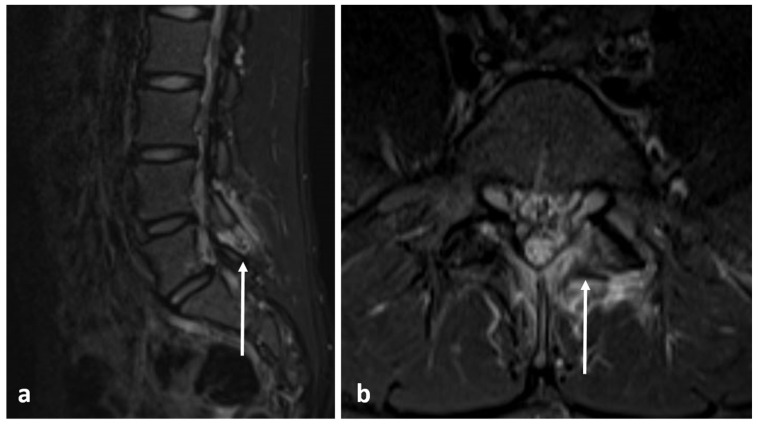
Sagittal (a) and axial (b) STIR images demonstrating the same osteoid osteoma showed in Figure 3a,b (white arrows). High STIR signal corresponding to oedema is typical. The nidus is not clearly seen in MRI.
